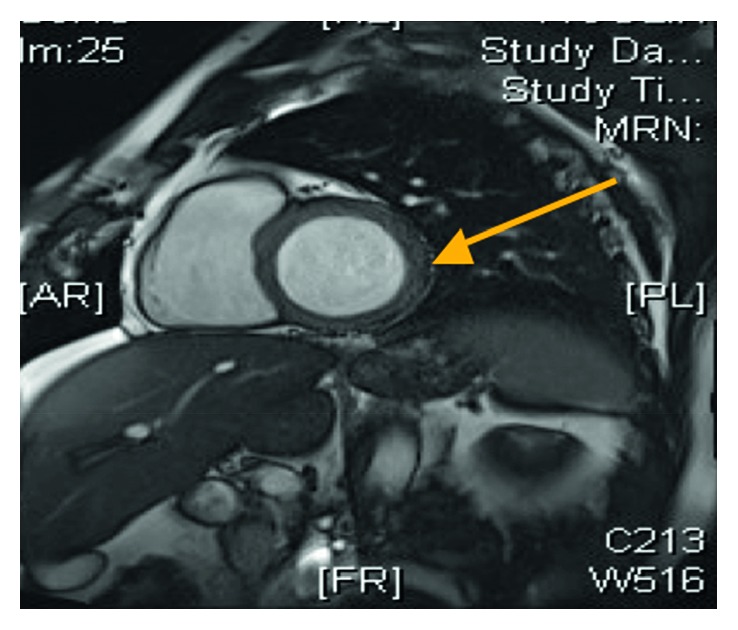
Short-axis view of the cardiac MRI demonstrating a normal left ventricular size and features consistent with myocarditis (arrow).
